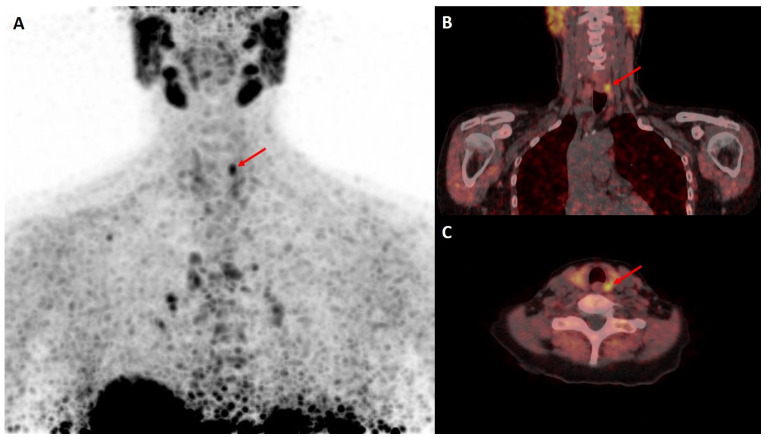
Imaging of a parathyroid adenoma, indicated by a red arrow, in a 74 year old female patient with elevated calcium levels using [^18^F]fluorocholine PET/CT, showing (A): maximum-intensity-projection PET, (B): coronal PET-CT and (C): transaxial PET-CT slices.
